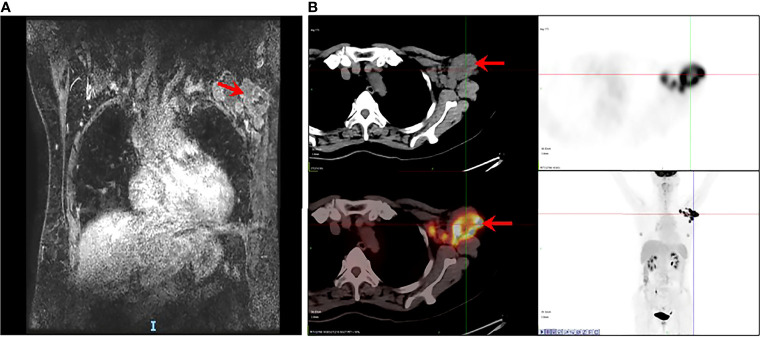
Occult breast cancer accompanied by metastasis in axillary lymph nodes. (A) MRI showing multiple enlarged and fused lymph nodes (arrow). (B) PET/CT showing multiple enlarged nodes on the deep muscles of the left axilla and the left anterior upper chest wall, radioactively concentrated (arrow) as well as with no evident metabolically active lesion in the bilateral breasts, remaining trunk, and brain.
